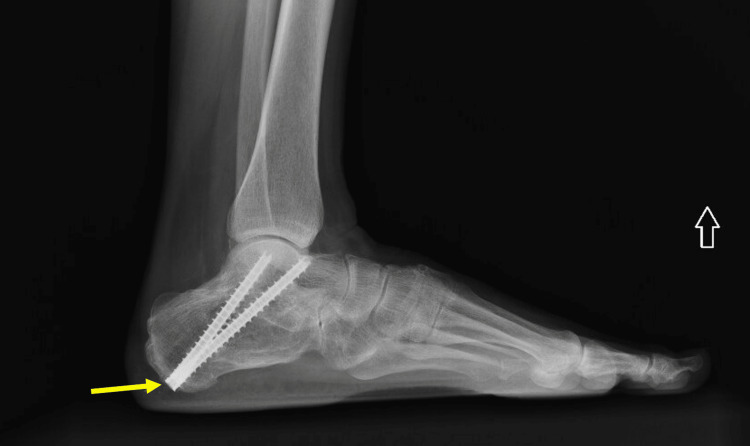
Left Ankle Lateral Plain Film Radiograph The planned surgery was the removal of the two screws (yellow arrow) in the left calcaneus.
